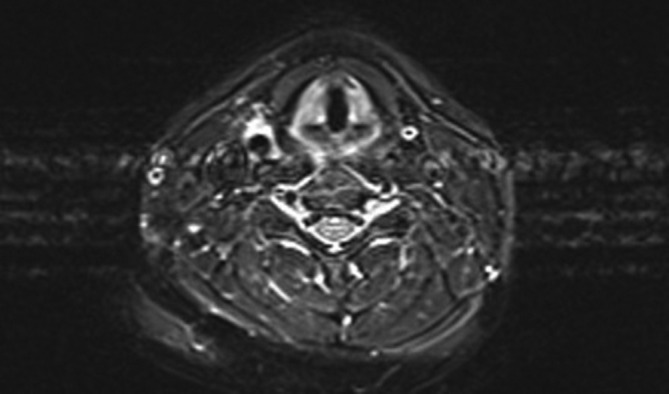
T*_2_ weighed MR image of the neck showing hyperintense perivascular thickening around distal right common carotid artery.
